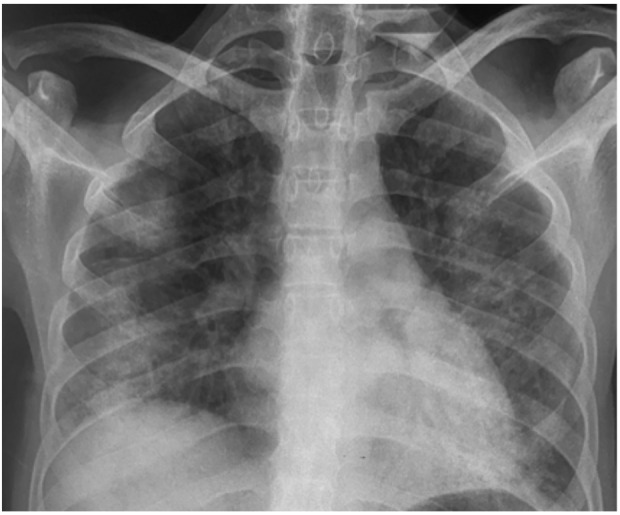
Chest radiograph showing Ground glass opacification in both middle and lower lungs zone
